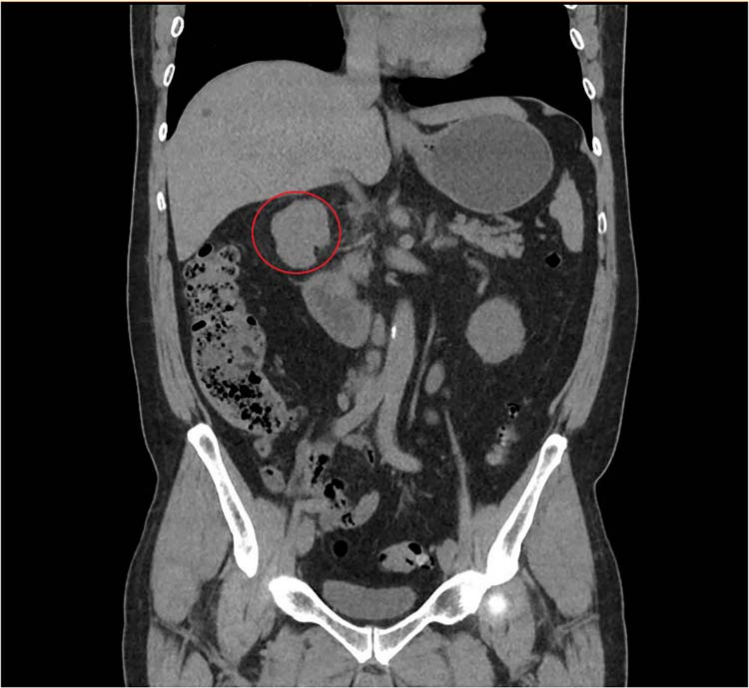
Coronal abdominal CT scan revealing a retroperitoneal polylobate neoformation near the duodenum.
